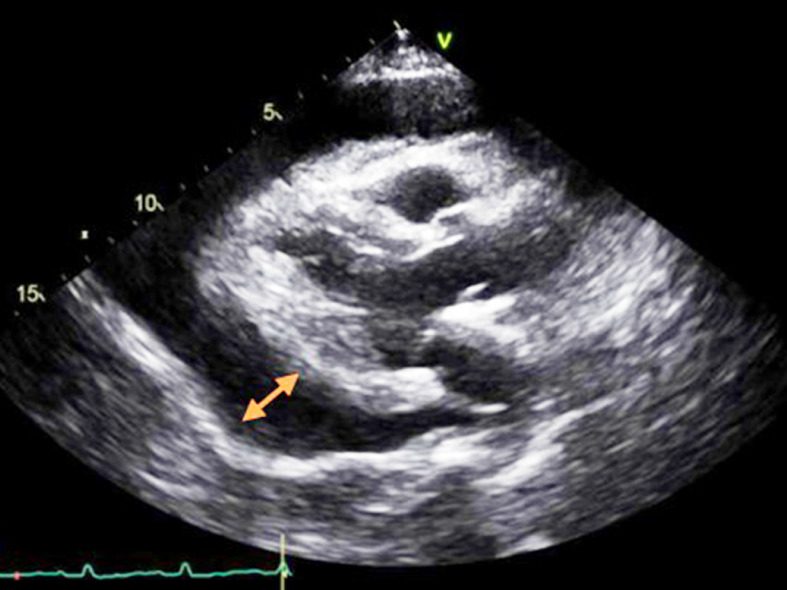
Transthoracic echocardiogram showing large pericardial effusion (orange arrow) with impending cardiac tamponade.
